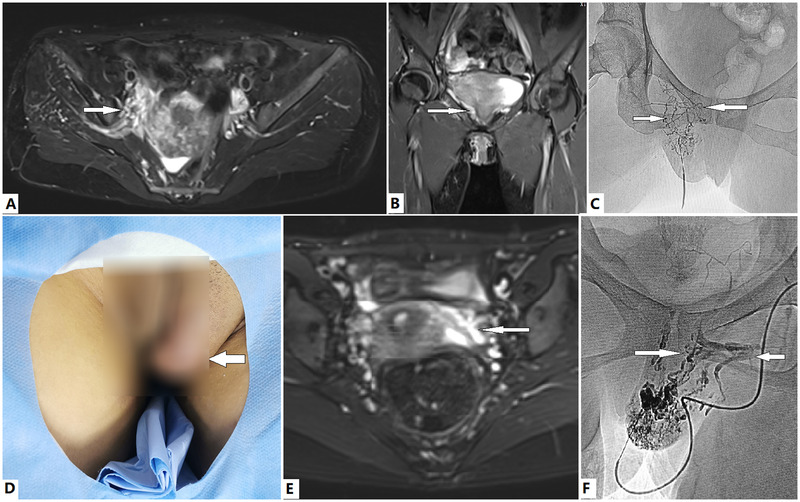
Evaluation and treatment of dysmenorrhea via direct puncture in female patients with Klippel–Trénaunay syndrome (KTS). (A–C) A 37-year old woman with KTS presented with progressive dysmenorrhea for several years. Dilated tributaries of the internal iliac vein, including the uterine veins, are identified by the T2 magnetic resonance imaging (T2 MRI) sequence (A,B, arrows). The malformed labial, vaginal, and uterine veins (C, arrows) were venographed, embolized, and sclerosed with ethanol via direct puncture of the dilated labial veins. Dysmenorrhea was successfully managed after two treatment sessions. (D–F) A 16-year old girl with KTS presented with progressive dysmenorrhea since menarche. The labial venous malformation (VM) is visible (D, arrow). Dilated incompetent uterine veins are revealed by T2 MRI sequences (E, arrow). A typical fluid signal in the lumen indicates flow stagnation and/or reflux. The labial VM, vaginal veins (F, rightward arrow), and incompetent uterine veins (F, leftward arrow) are venographically embolized and sclerosed with ethanol via direct puncture of the labial VM. Complete relief of dysmenorrhea was obtained after one session of treatment. However, the labial VM requires further management.
